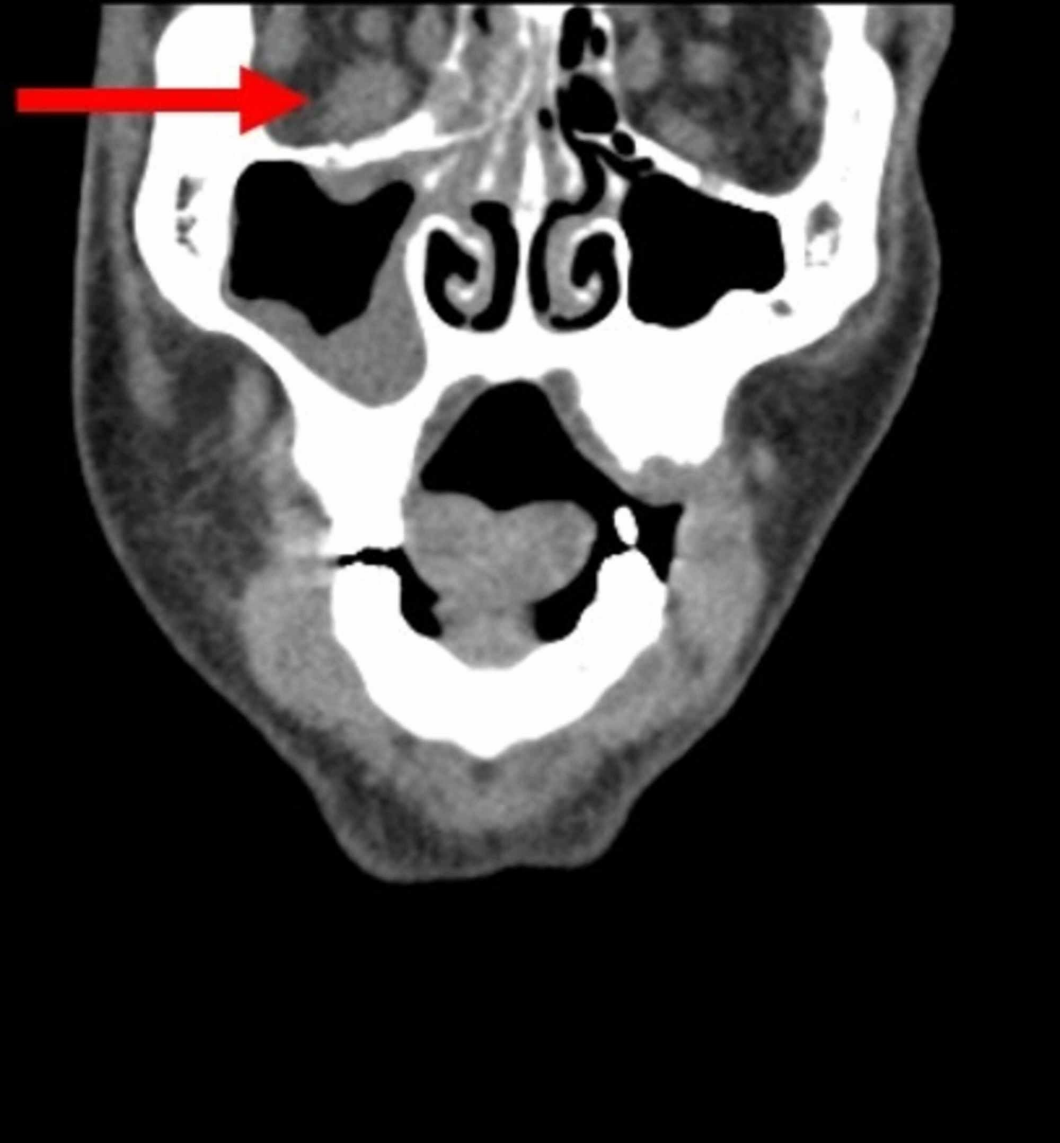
Coronal non-contrast CT on admission. The red arrow shows marked enlargement and indistinctness of the right inferior rectus muscle indicating inflammatory changes.
